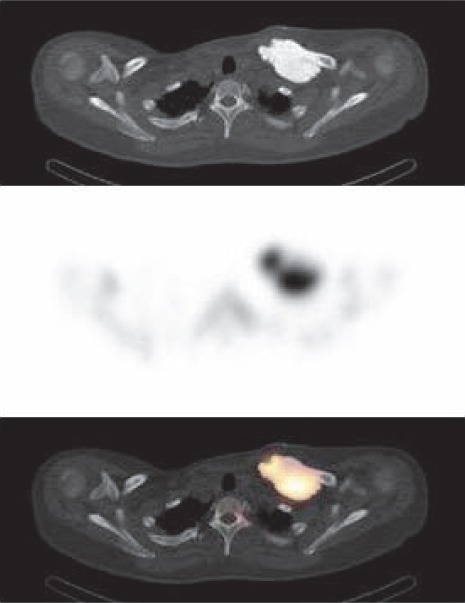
The transaxial CT image (upper section) identifies a dense lesion with well-defined contours in the left clavicle. There is intensely increased uptake on the corresponding SPECT image (middle section) and SPECT/CT fusion image (lower section)
